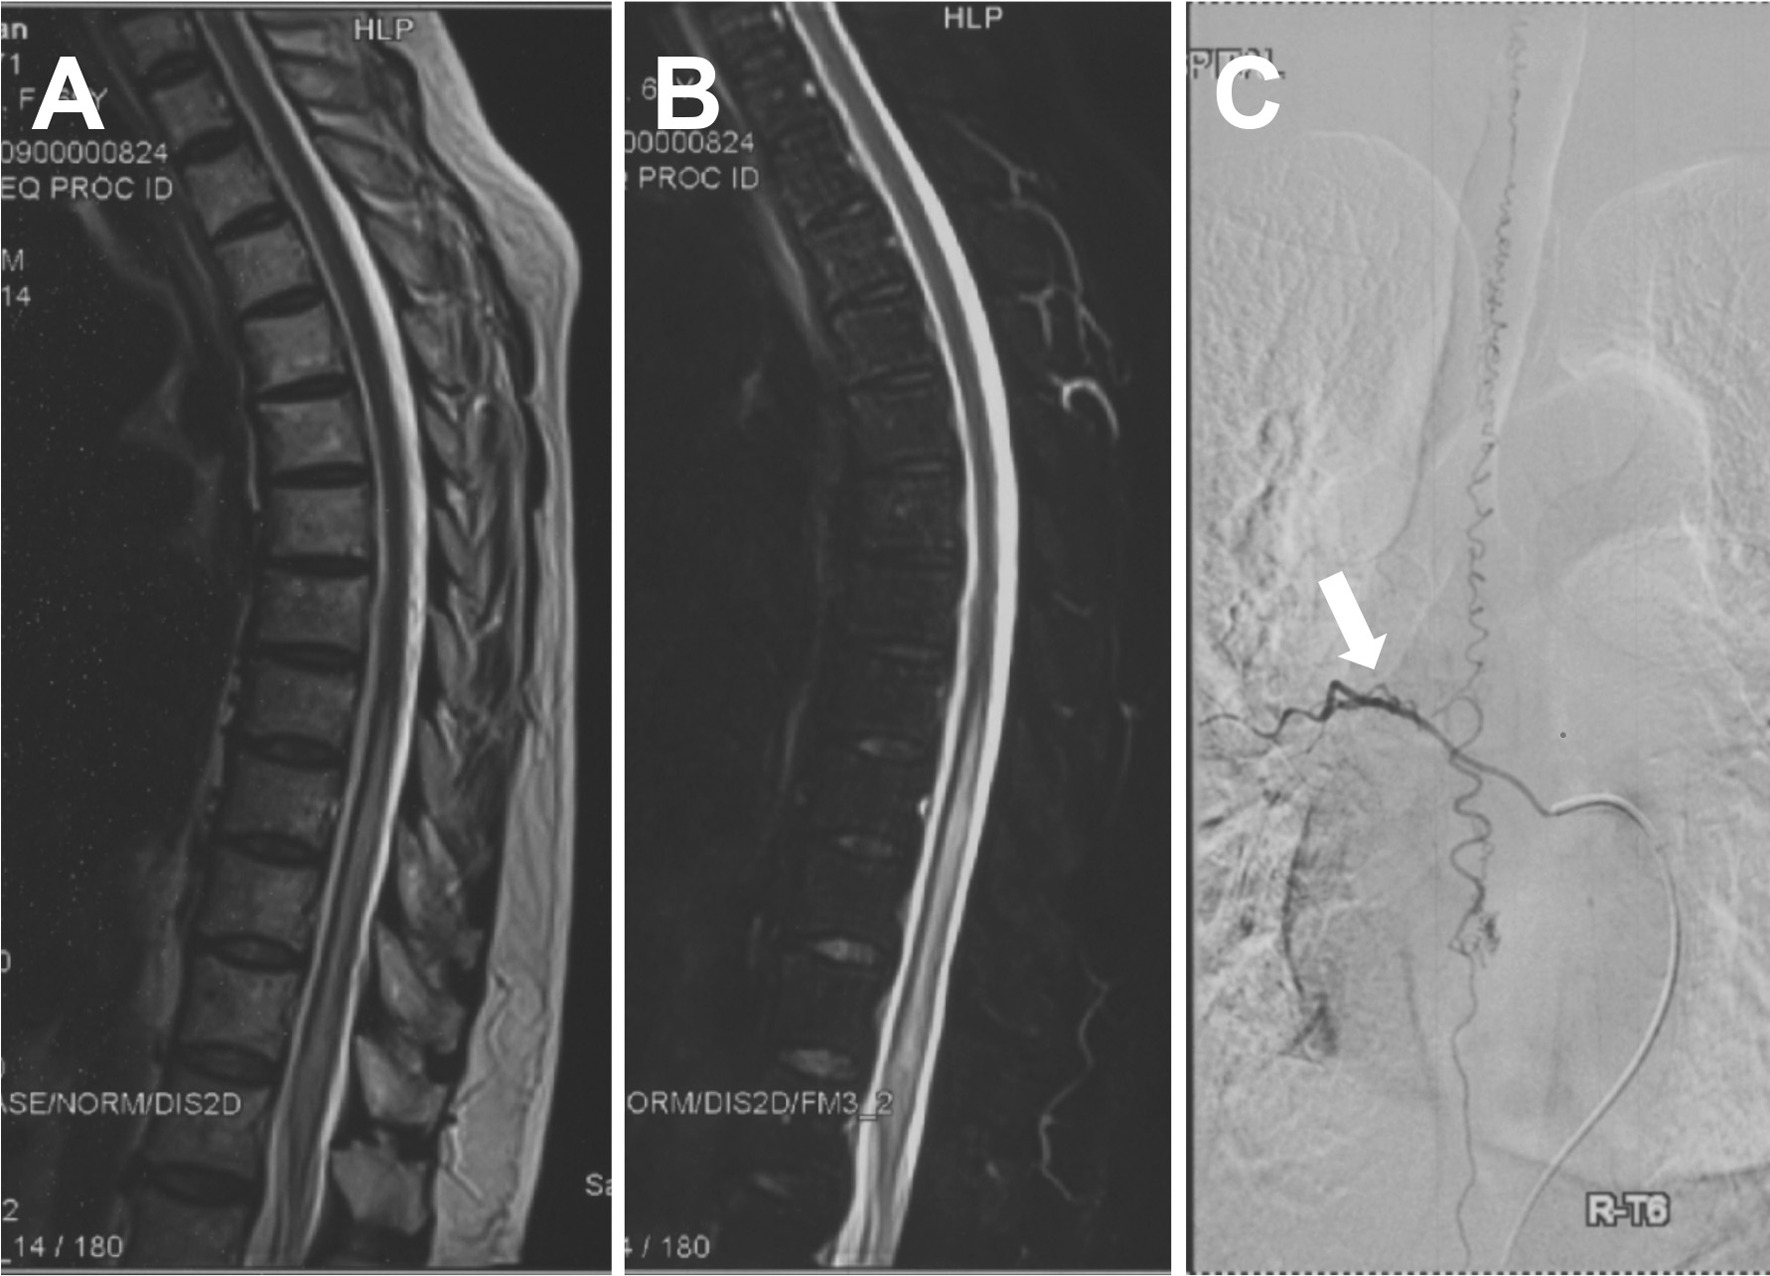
Imaging findings of a patient with misdiagnosis. A 69-year-old woman complained of lower-extremity progressive weakness and numbness over one year. Magnetic resonance imaging showed a multisegmental intramedullary T2 hyperintensity (A) with diffuse contrast enhancement (B). There was no evidence of obvious fluid voids. C DSA revealed a fistula (white arrow) at the right T6 level, fed by the radicular artery rising from the segmental artery
